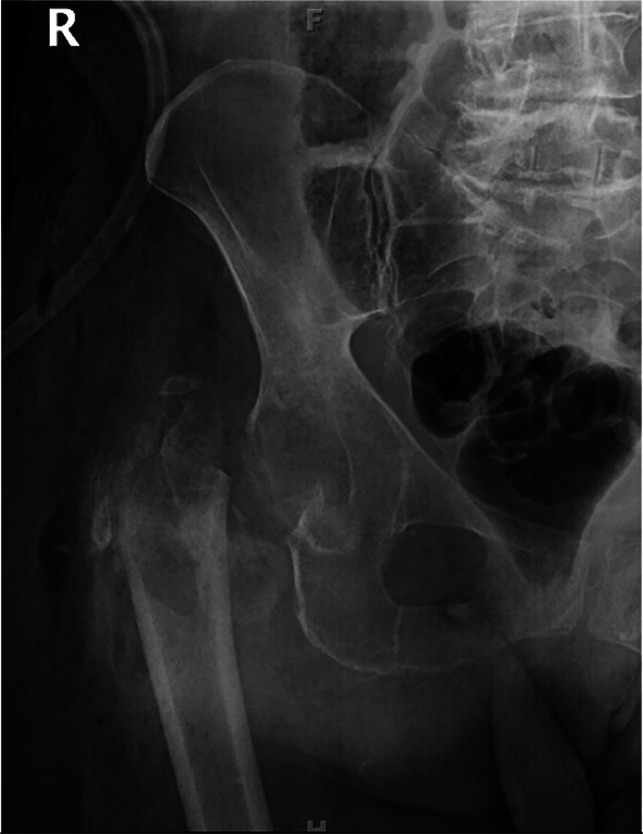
Radiograph showing examples of proximal femoral bone loss with absent bony landmarks
